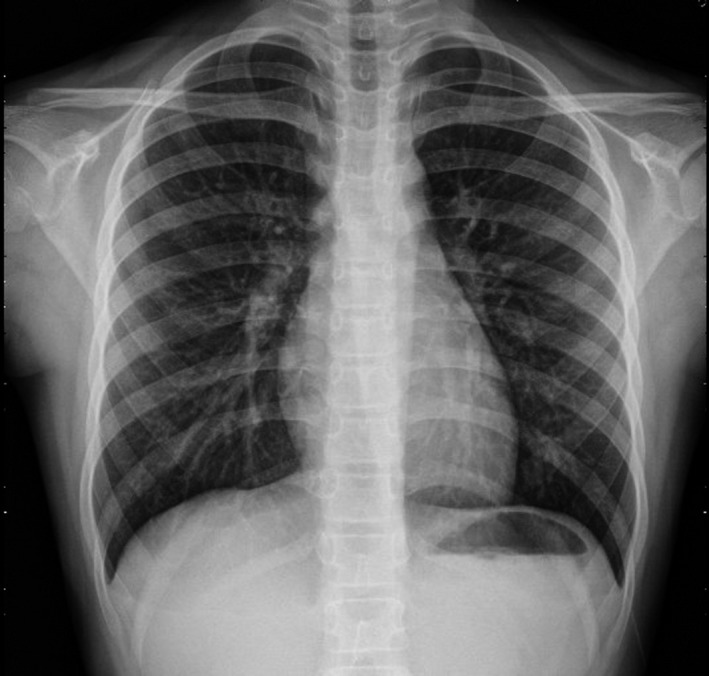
Chest radiography of the patient on first day of admission. Slight increase observed in markings in bilateral lungs.
